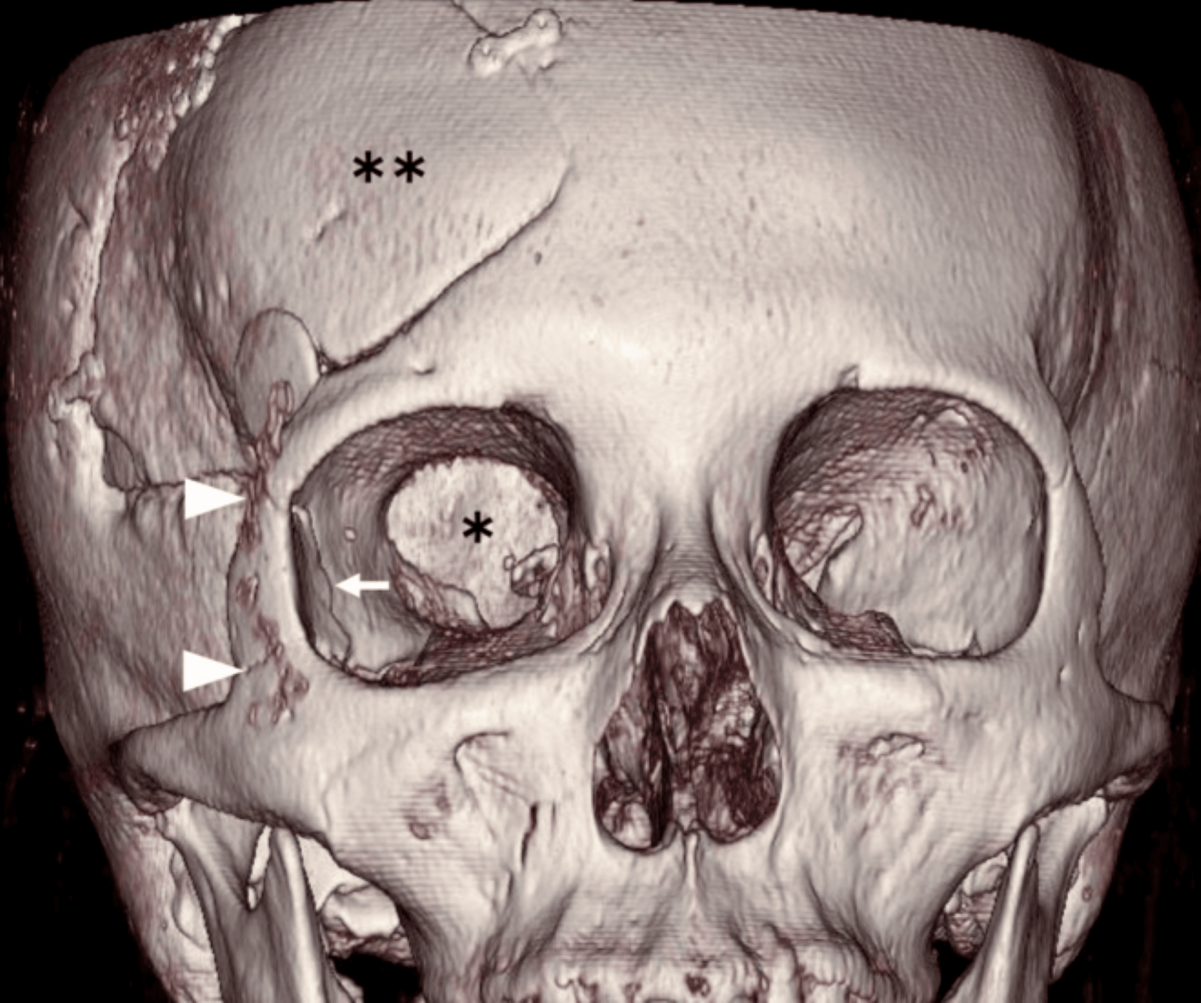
Postoperative three-dimensional CT image of the skull The lateral orbital rim was reconstructed using two resorbable plates without defect of the lateral wall of the right orbit (arrow). Note that after a previous craniotomy, the bone flap (double asterisks), titanium plates, and a ceramic button covering the keyhole were shown, and the superior orbital fissure (asterisk) was enlarged. Arrowhead osteotomy line of the lateral orbital rim in lateral orbitotomy.
